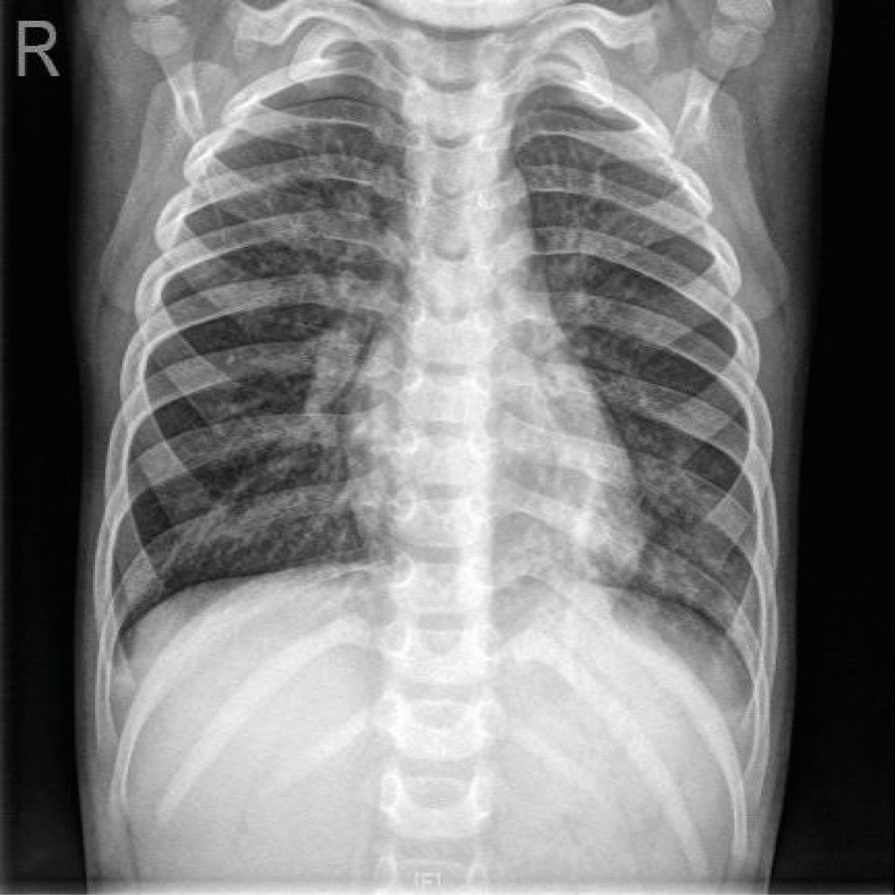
After bronchoscopy, a follow-up chest radiograph:the left lower lobe consolidation was significantly absorbed
